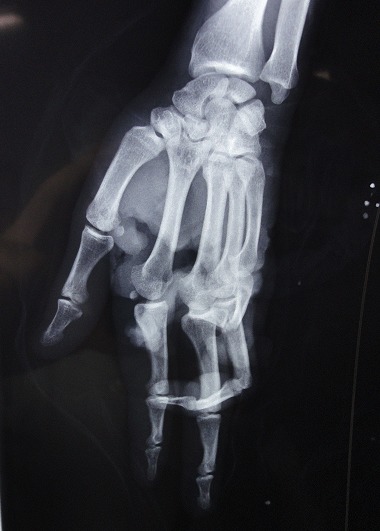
Radiograph of the right hand showing distal interphalangeal joint dislocation of the 5th finger
